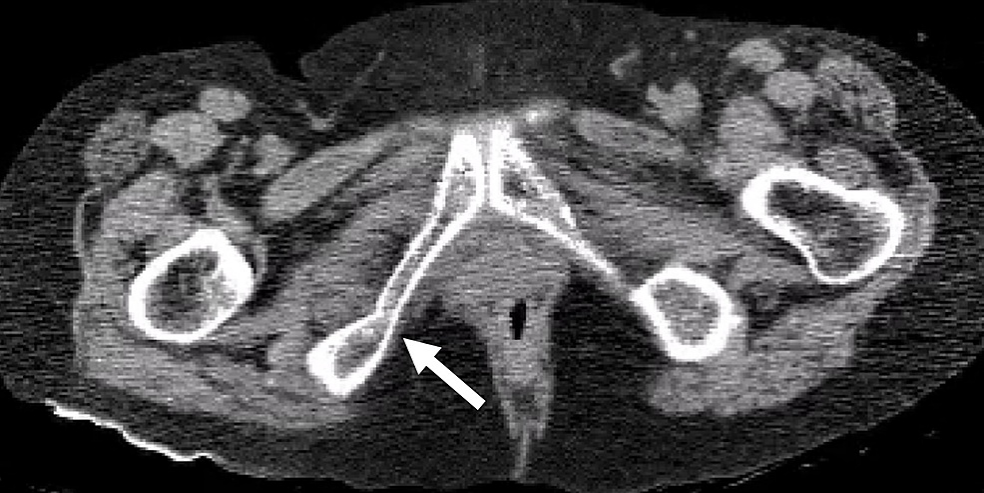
CT findings on arrival CT showed a right minor ischial fracture (arrow) CT: computed tomography
